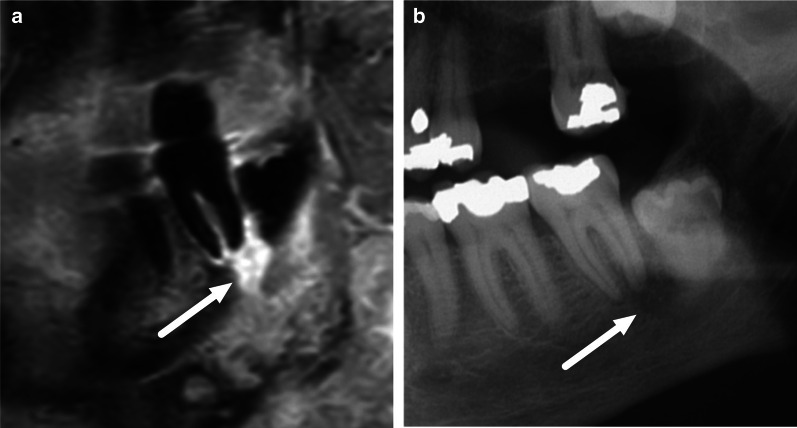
Periapical enhancement (arrow) at the tooth responsible for the neck infection, on a sagittal fat-suppressed post-contrast T1-weighted image (a). Radiography showed corresponding periapical radiolucency (arrow) consistent with an infection (b)
